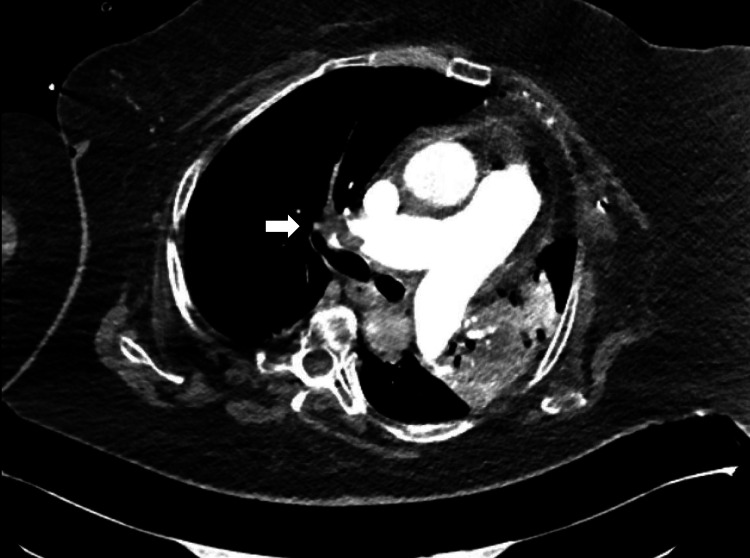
CTPA demonstrated a large right-sided pulmonary embolus (white arrow). CTPA: computed tomography pulmonary angiogram
